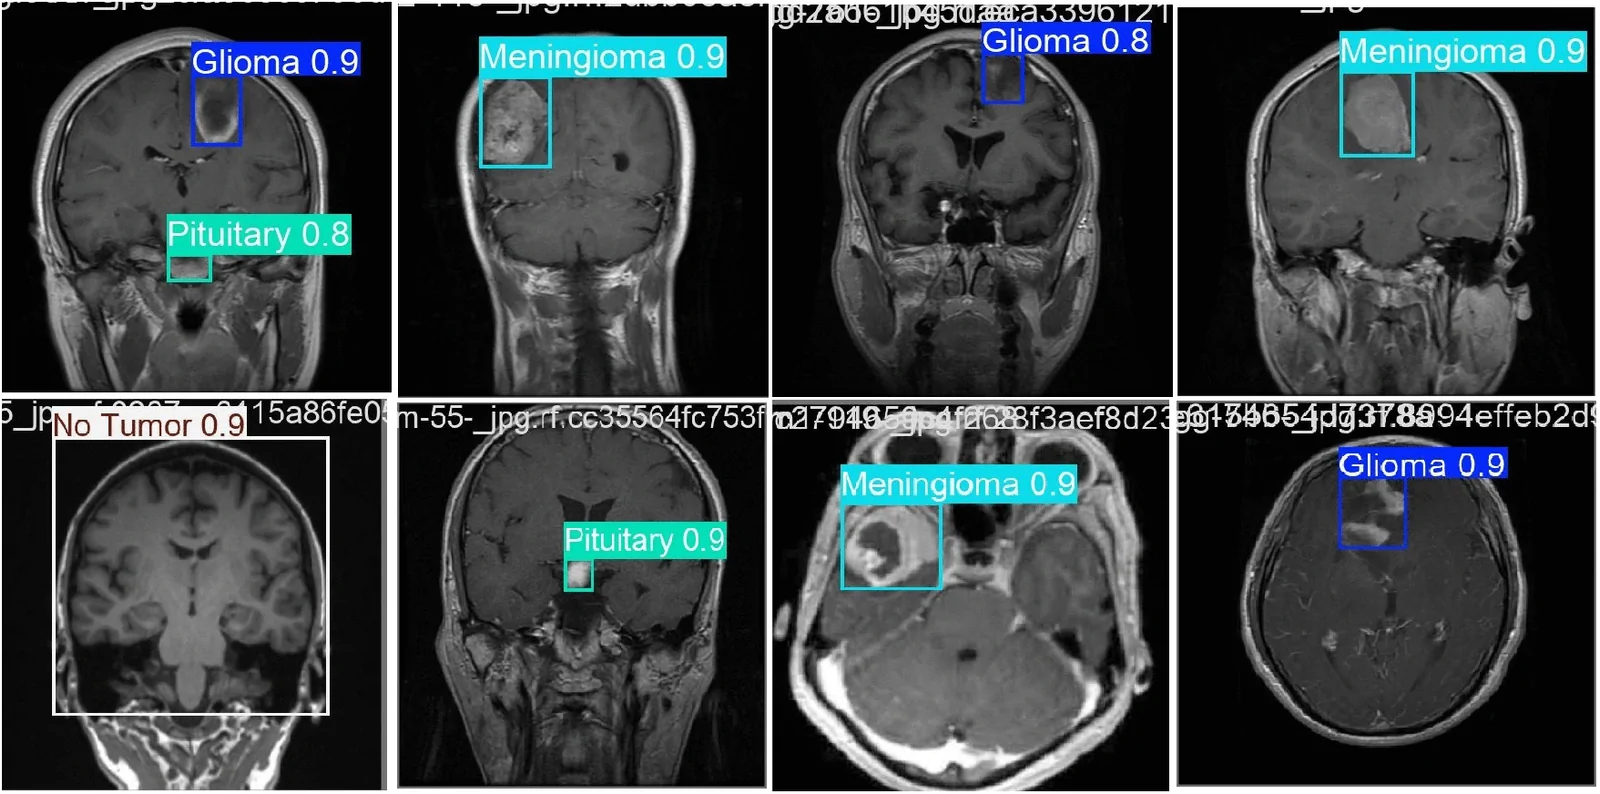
YOLOv11s prediction results. The underlying base MRI scans are obtained from the “Brain Tumor MRI” open-source dataset by workspace research-jz5sv [21] on Roboflow Universe, used under the CC BY 4.0 license.
